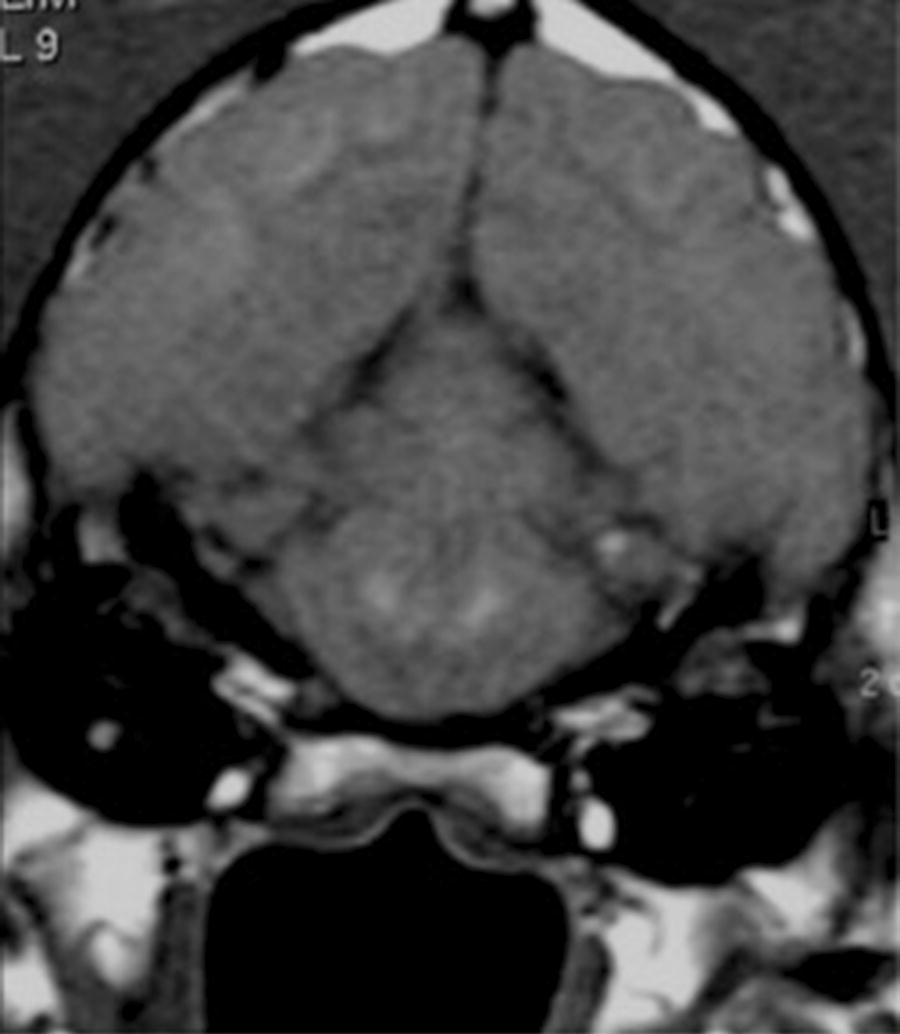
Brain MRI coronal T1 weighted image (post contrast) revealing enhancement in paracentral pontine lesions
